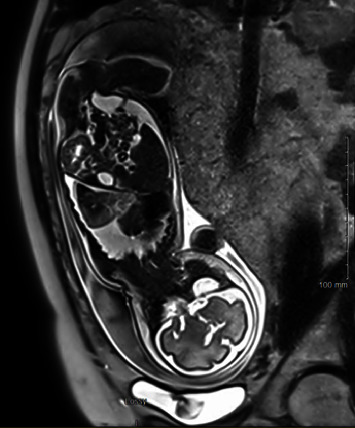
Foetal MRI at 27 weeks of gestation +3 days showing a 44 mm × 22 mm  ×  38 mm mass in the left lower lung lobe with an anomalous systemic vessel of 3 mm of diameter originating from the proximal part of the abdominal aorta, with concomitant major hydrothorax, right mediastinal shift and mild ascites.
